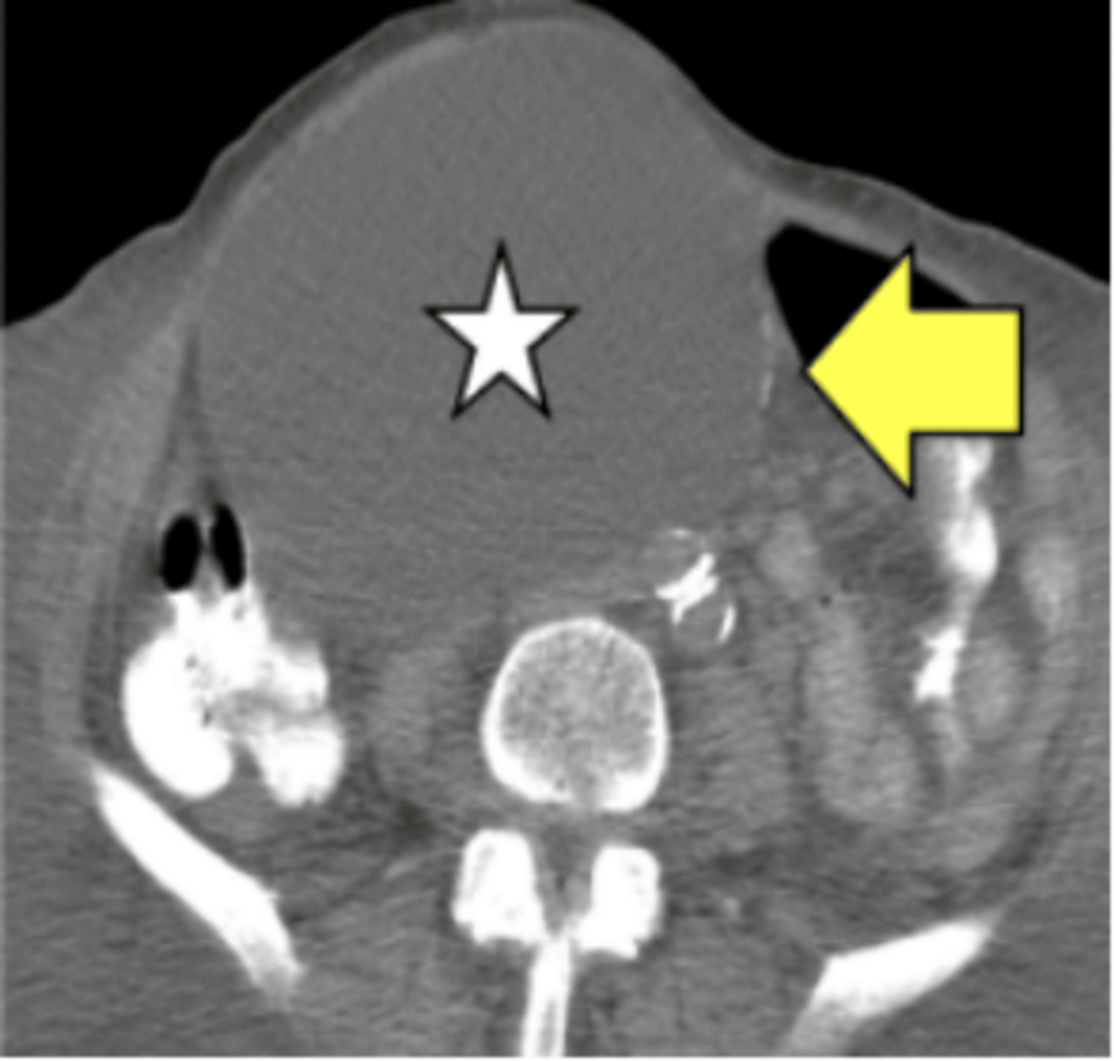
Mesenteric cyst. CT image shows a mesenteric cyst (white star) with a rim of calcification (yellow arrow).
